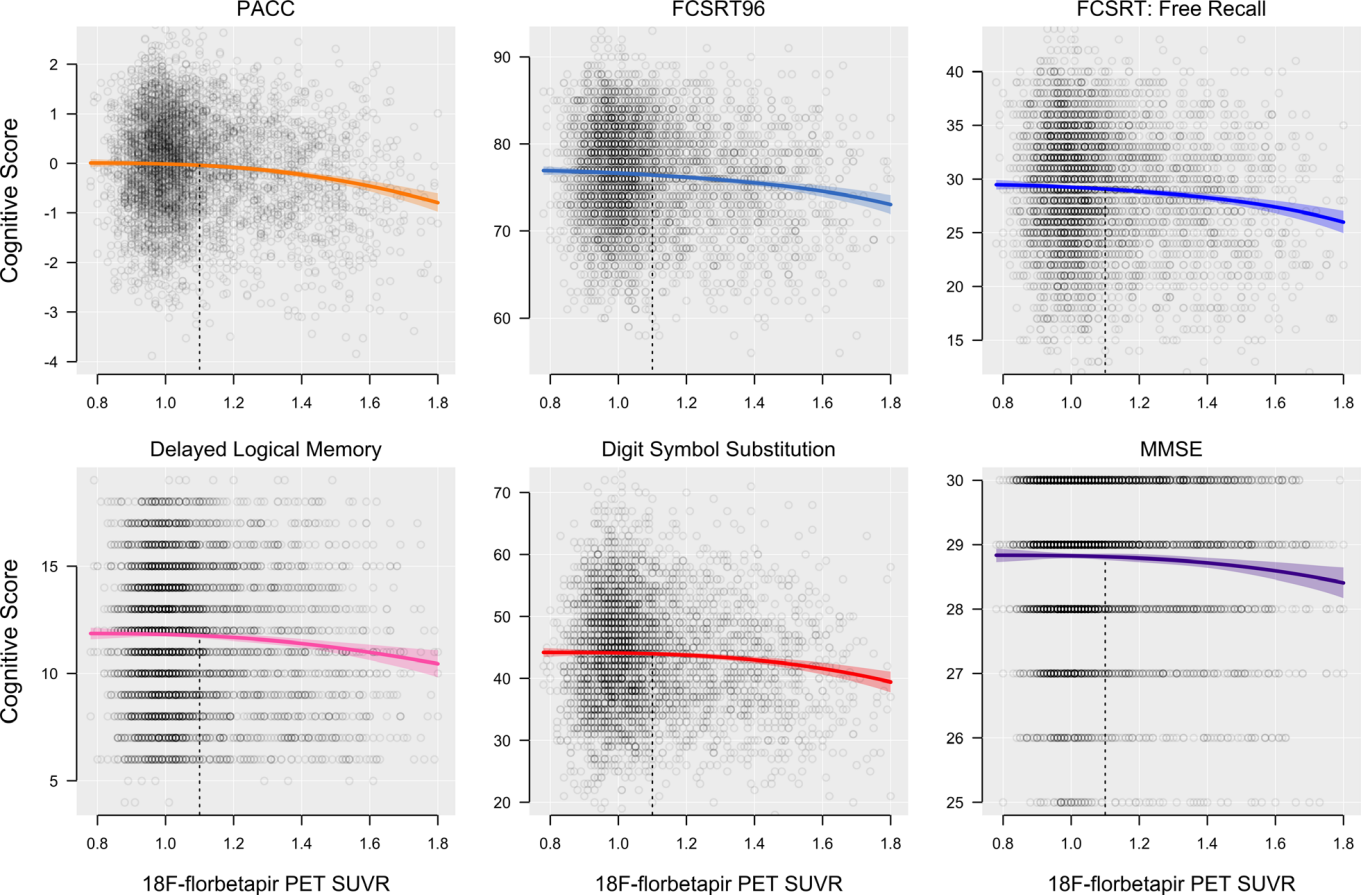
Effects of amyloid PET on cognition. Cognitive scores are plotted against 18F‐florbetapir PET SUVR with mean curves and 95% confidence intervals represented by the shaded region. The vertical dashed line indicates the threshold for A*β*‐positivity (SUVR = 1.10).
